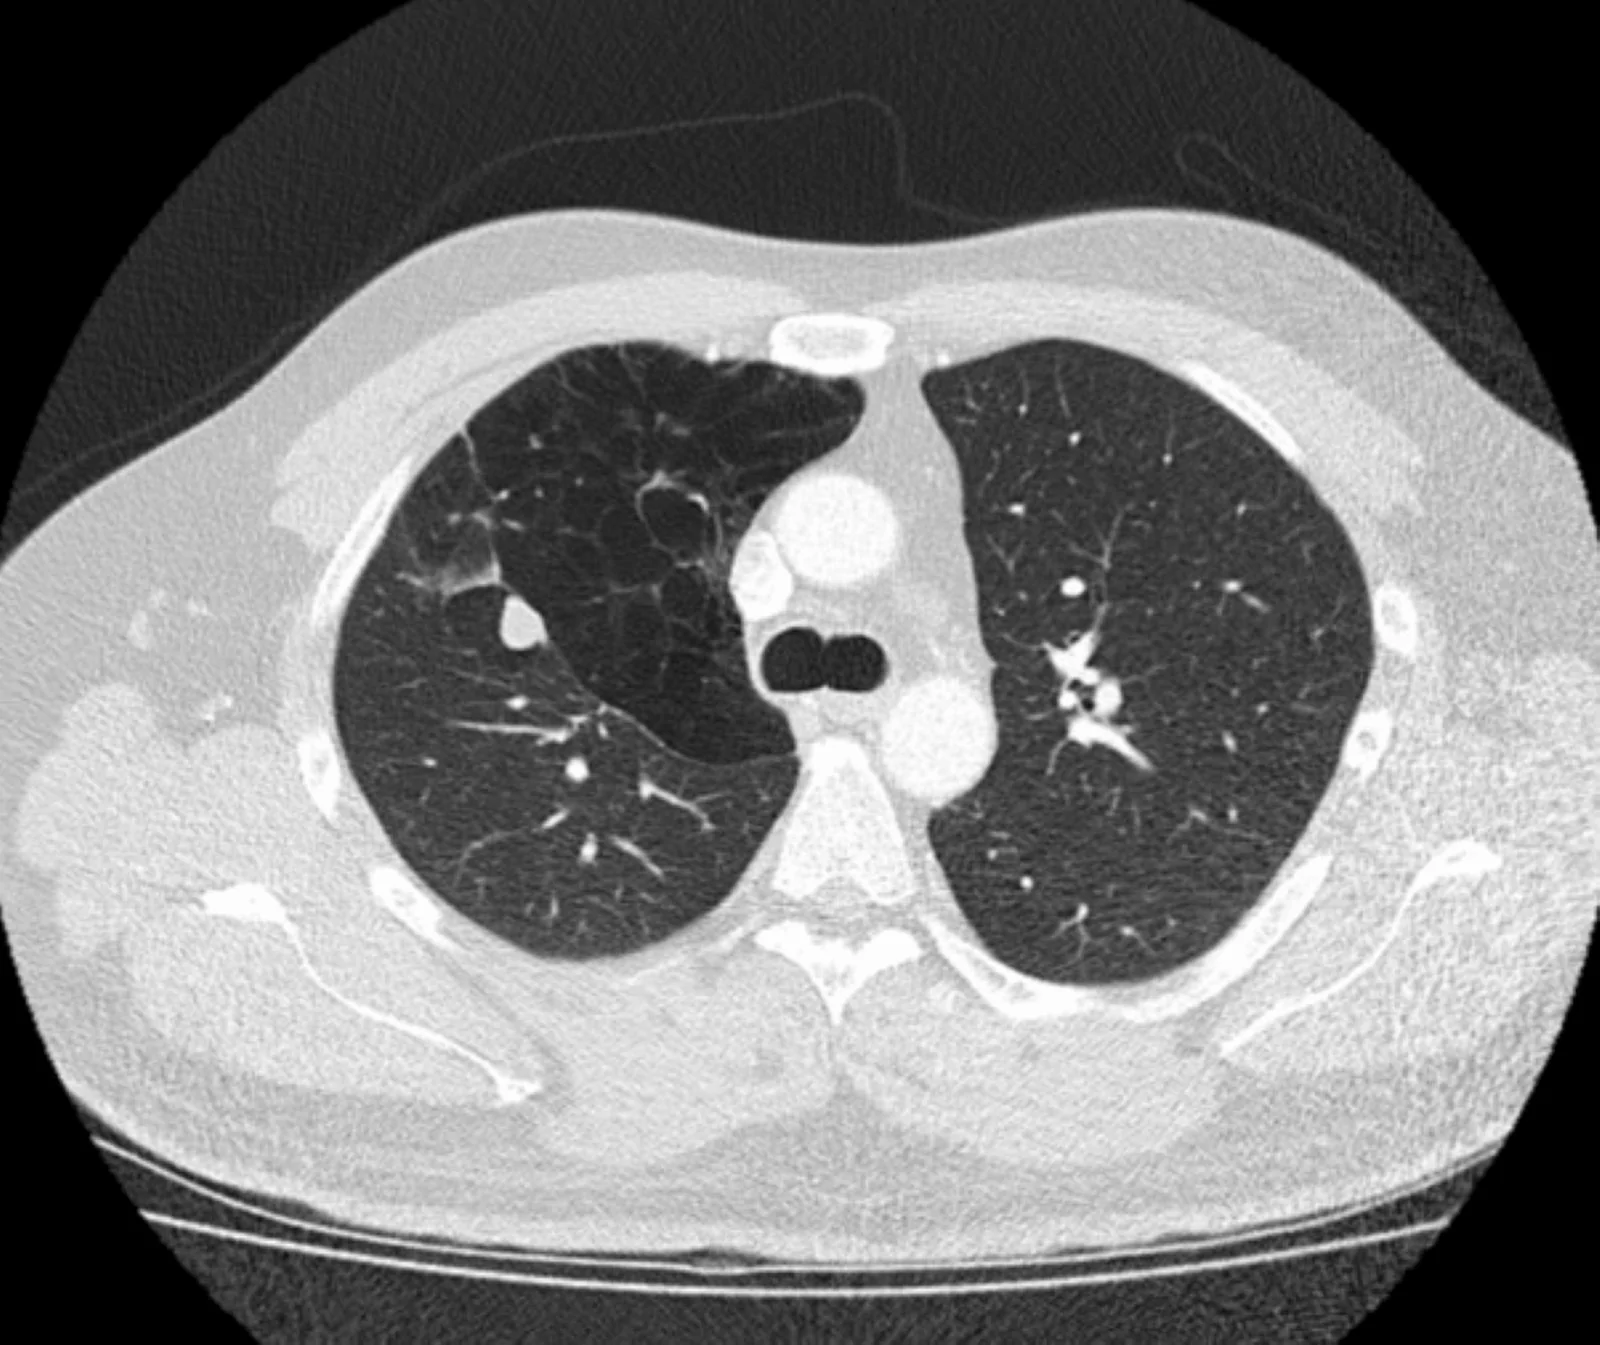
Axial contrast-enhanced CT scan demonstrating a large multicystic lesion of the right upper lobe.
